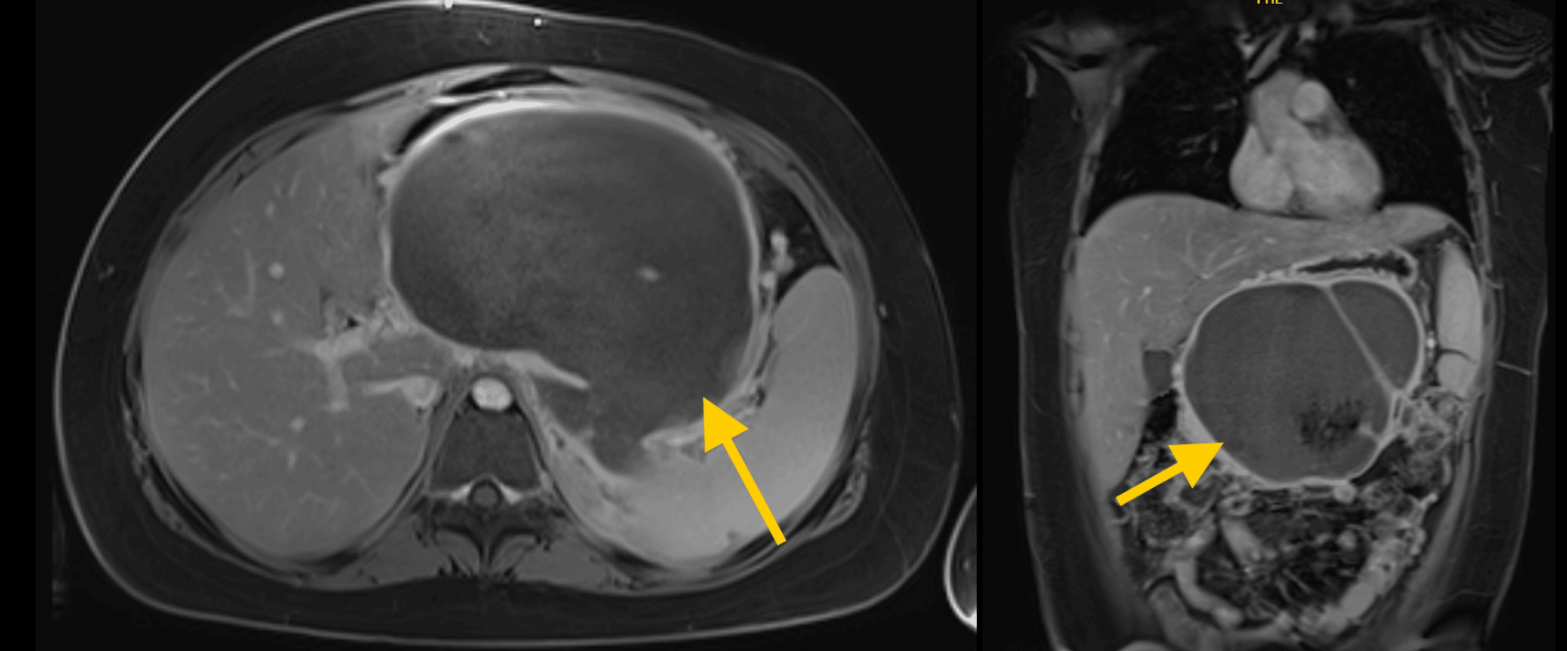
T1-weighted contrast enhanced MRI abdomen images axial view (left), coronal view (right) showing a large WON collection. WON: walled-off necrosis
